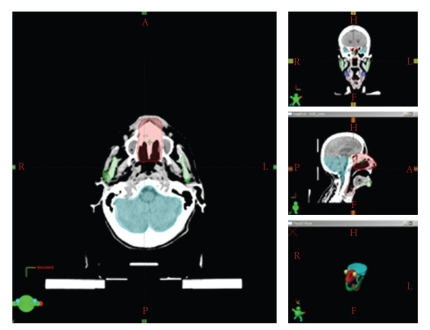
Radiotherapy planning II*. Another CT section at the lower PTV boundary. Small insets show the complete extension of the PTV.
